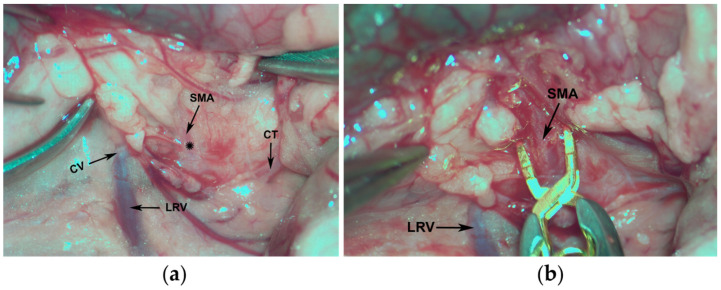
View of the surgical field: (a) anatomical location of the main structures: CV (cava vein), LRV (left renal vein), CT (celiac trunk), and SMA (superior mesenteric artery), the asterisk indicates the site of placement of the microvascular clip; (b) detail of the dissected SMA with the microvascular clip.
